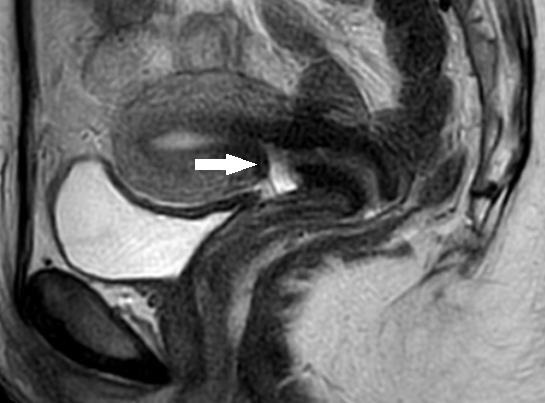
Preoperative MRI imaging. Legend: arrow indicating niche.
